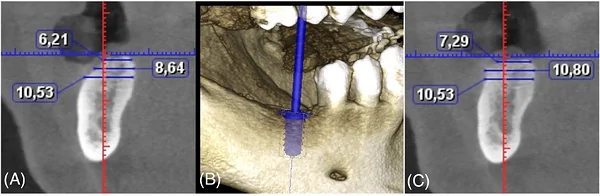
Cone beam computed tomography (CBCT). (A) Baseline measurements made at 1, 3, and 5 mm beneath the bone crest. (B) 3D representation revealed bony dehiscence if no bone augmentation was performed. (C) 5MO follow‐up measurements made at 1, 3, and 5 mm beneath the bone crest showing an increase in ridge width.
